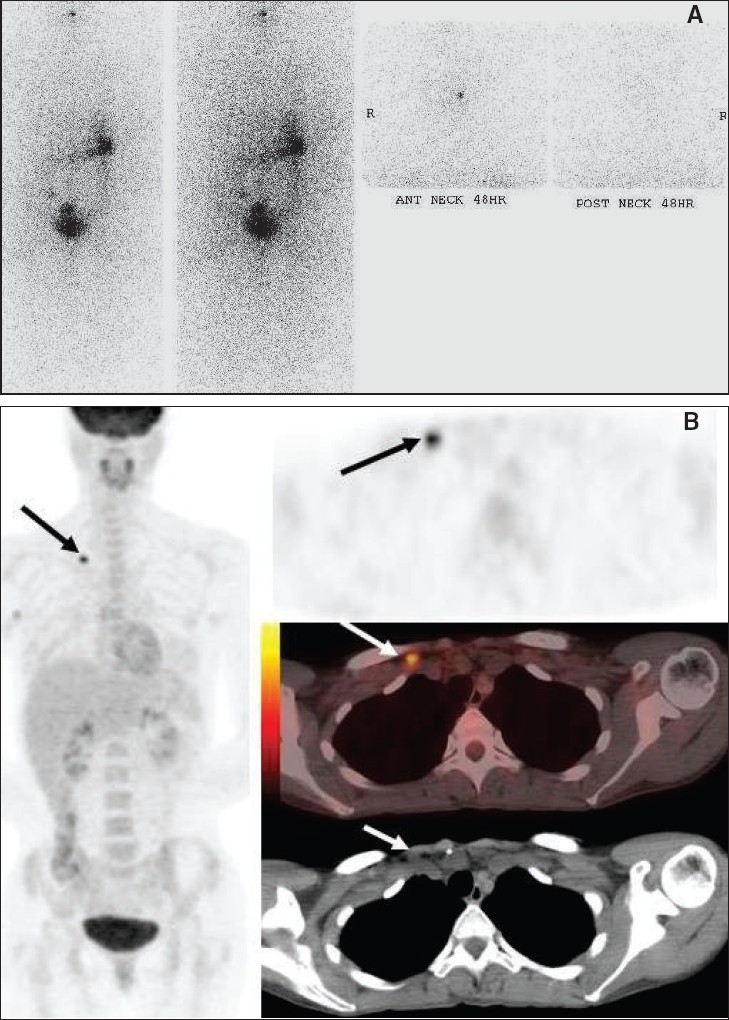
A 32-year-old lady with a history of pT4a, pN1b, Mx papillary thyroid cancer treated with surgery followed by radio-ablation with 200 mCi of I-131 with no I-131 avid metastases on the 7-day post-treatment scan. She continued to have elevated thyroglobulin levels; but physical examination, USG, CT scan and repeat imaging with I-131 whole-body scan (A) failed to reveal evidence of residual/recurrent disease. Subsequent PET study (B) with axial PET (top right), fused PET/CT (middle right) and CT scan (bottom right) images at the level of the supraclavicular fossa, with an MIP image (on left), showed an intense right supraclavicular node (arrows), measuring 1.0 cm in diameter, which was proven to be metastatic thyroid cancer
